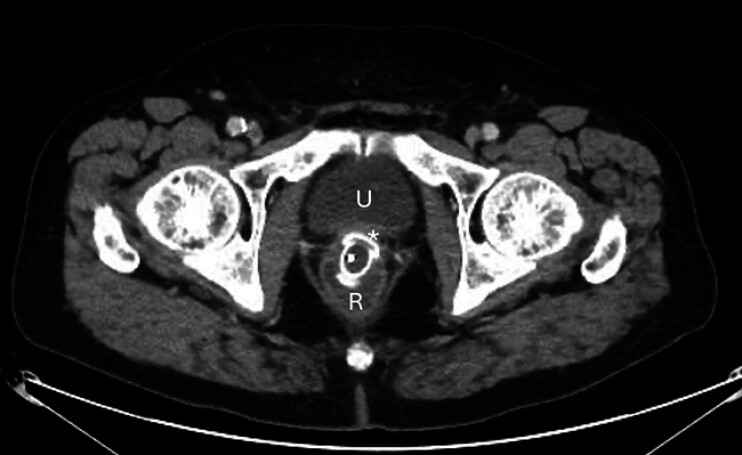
Computed tomography image two weeks after drainage, showing the lumen apposing metal stent connecting the residual abscess cavity (asterisk) with the rectal stump (R). “U” denotes the urinary bladder.
